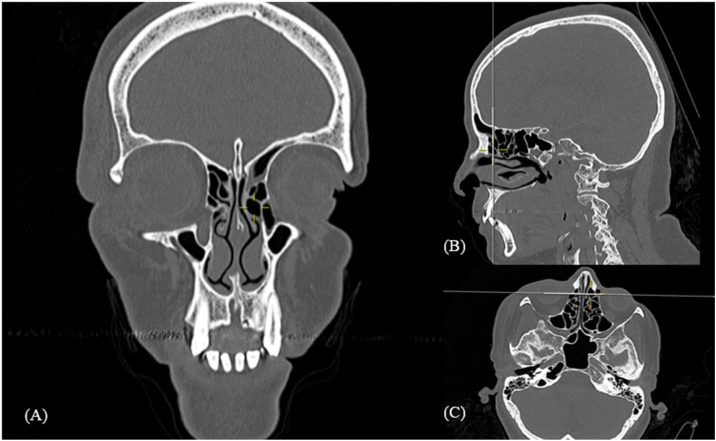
Agger nasi cell. Computed tomography: Mark shows left side agger nasi cell in coronal (A), sagittal (B) and axial (C) reconstruction.
